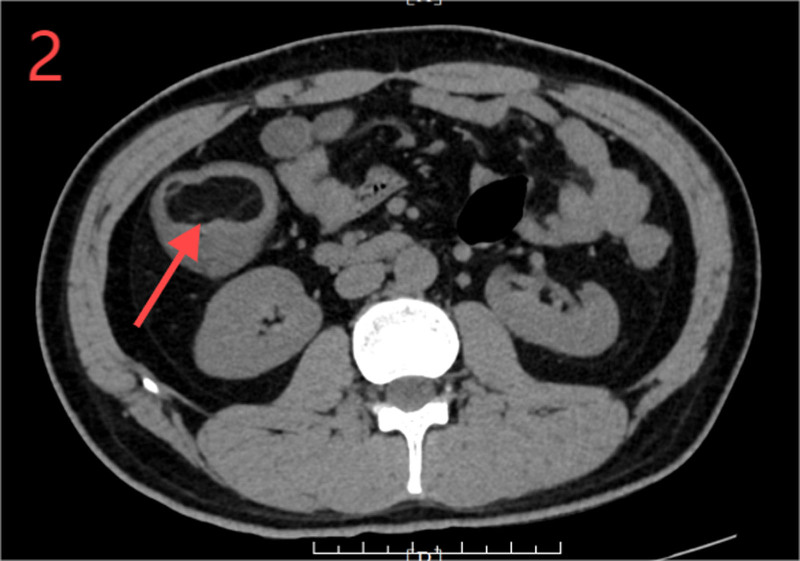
Computed tomography image (transverse plane) shows a circular fat density mass in the lumen of the ascending colon.
